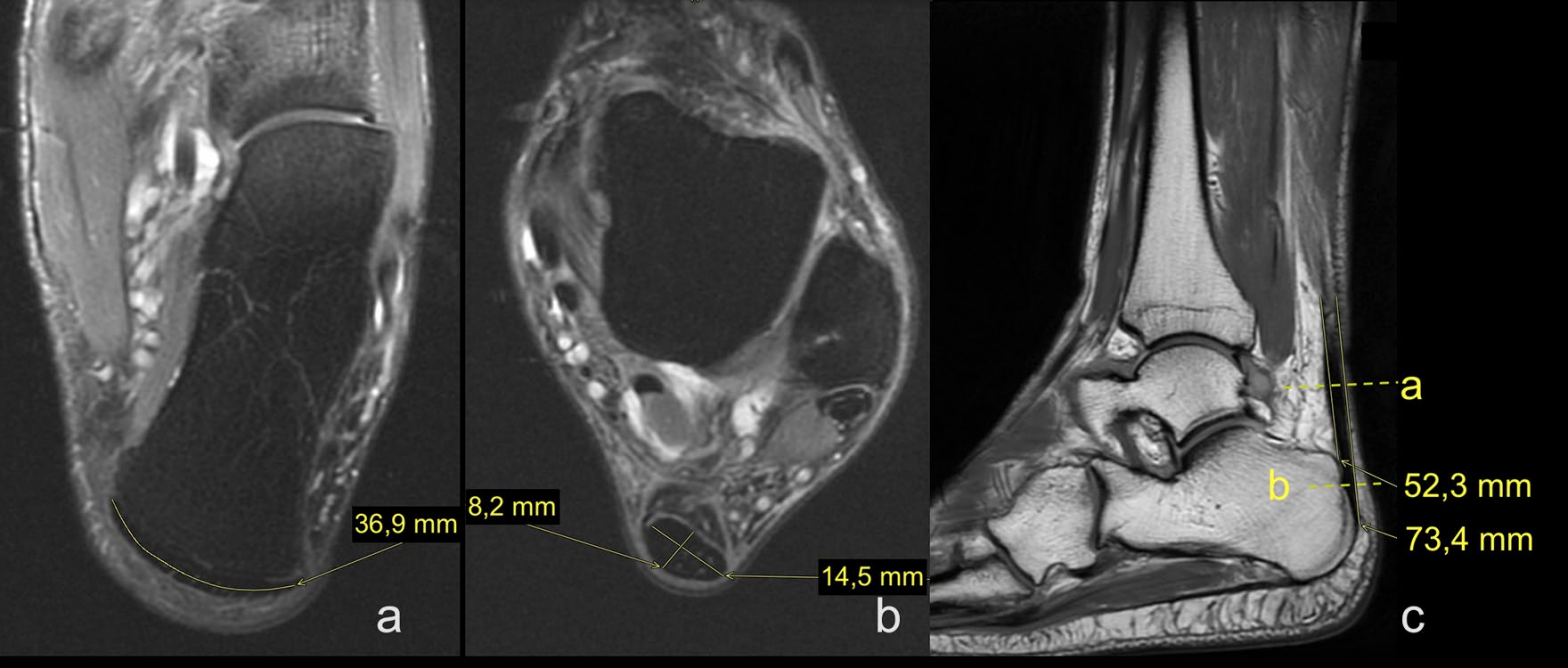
Diameters of the normal Achilles tendons in two patients. (a) and (b) a 34-year-old female with clinical suspicion of enthesopathy of the Achilles tendon, (c) a 28-year-old male with clinical suspicion of myositis. (a) and (b) axial section, PD-weighted FS, (c) T1-weighted.
